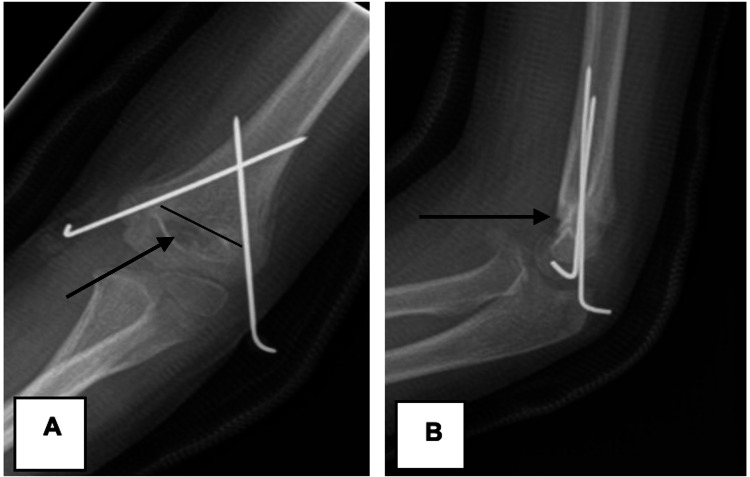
Radiographic example of adequate fixation using the crossed K-wire technique (A) Anteroposterior and (B) lateral radiograph showing adequate reduction and fixation of the displaced supracondylar fracture with crossed wires (bi-cortical fixation with adequate separation at the fracture site).
